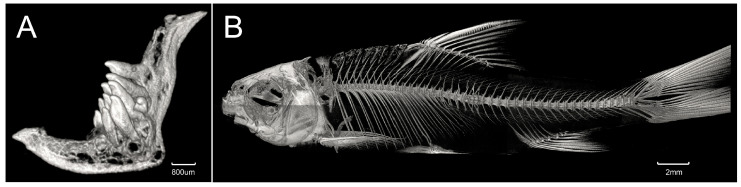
Pharyngeal dentition (A) and vertebrae (B) of the CT scan of *Hongiastoma zhangbuensis* sp. nov. paratype, KIZ 2024011625, 110.6 mm SL.
